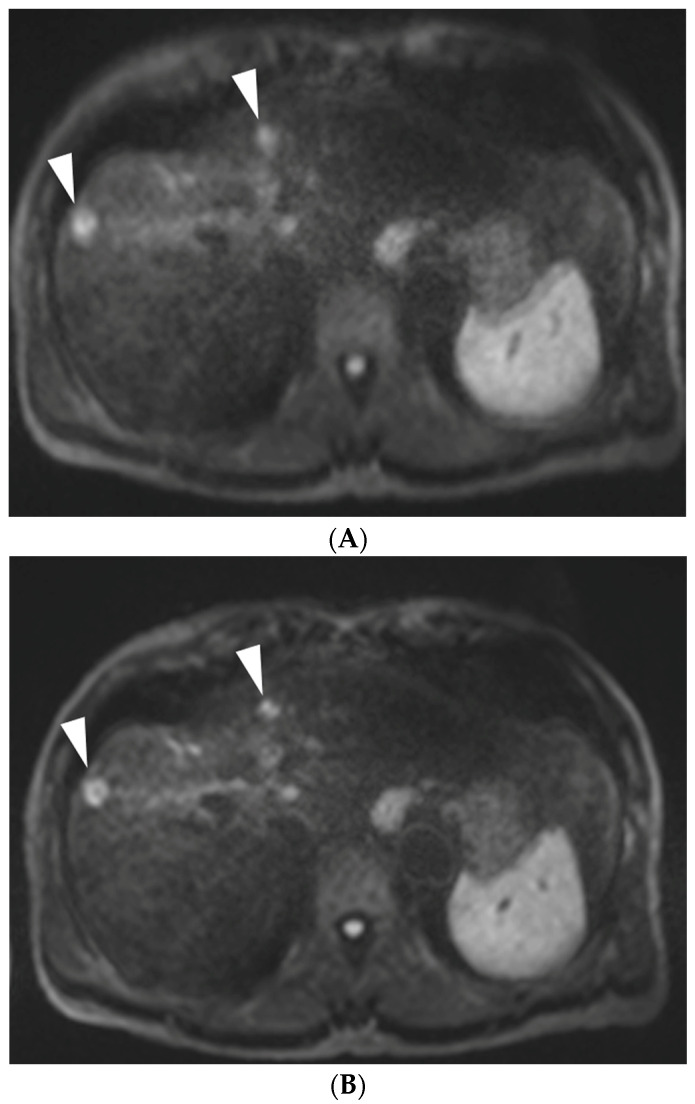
A 57-year-old man with hepatocellular carcinomas who underwent DWI. (A) Axial c-DWI (b = 800 s/mm^2^) and (B) axial moco-DWI ((b = 800 s/mm^2^). The conspicuity of the two hepatocellular carcinoma nodules (arrowheads) is better in moco-DWI (B) than in c-DWI (A).
